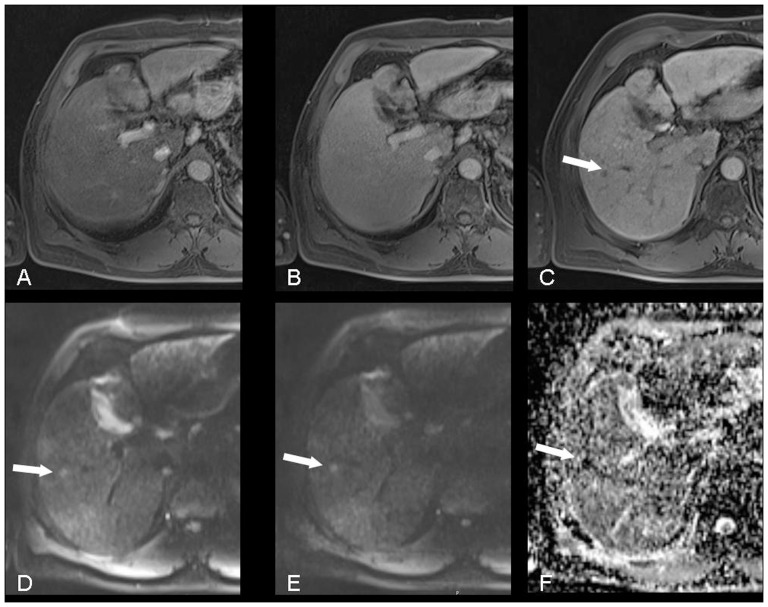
MRI of a 53-years-old male patient with HCC (arrow) in Segment 5: no lesion was identified in the arterial (A) and equilibrium phases (B), a small hypointense lesion was only observed in the hepatobiliary phase (C) and in DWI, where it is seen as a hyperintense lesion in b 50- (D) and b 400-images (E) and hypointense in the ADC-map (F).
